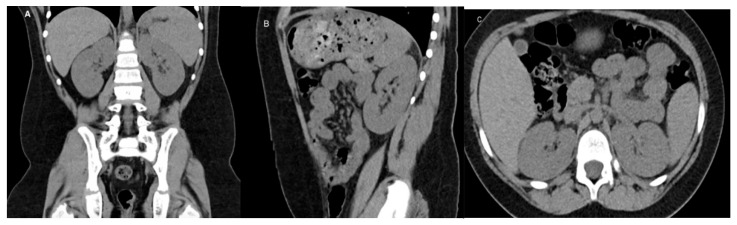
CT of a normal 8-year-old boy: (A) Coronal image, (B) Sagittal image, (C) Axial image.
